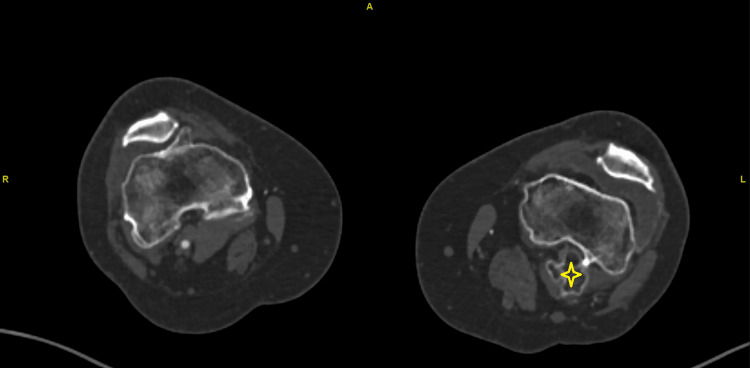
CT slice showing a large left femur exostosis. CT: computed tomography.
